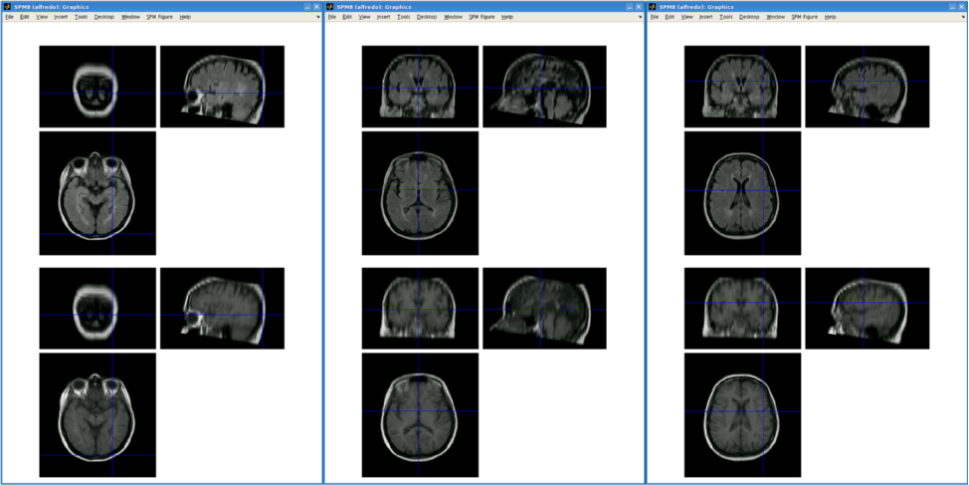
A visual coregistration check example using Check Reg tool in SPM. Left to right are presented three panels showing the output of Check Reg in case of a T1 (lower images) to FLAIR (higher images) sequences coregistration. Three relevant points were selected in order to check coregistration visually
